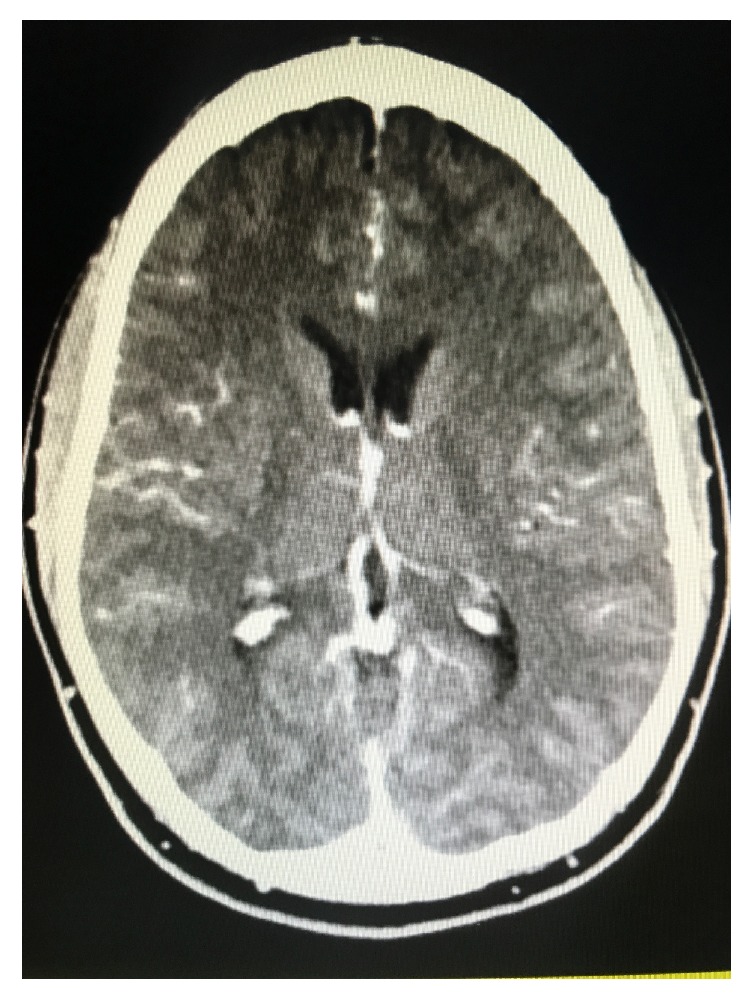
CT Scan showing diffuse inflammation and thickening of the meninges.
